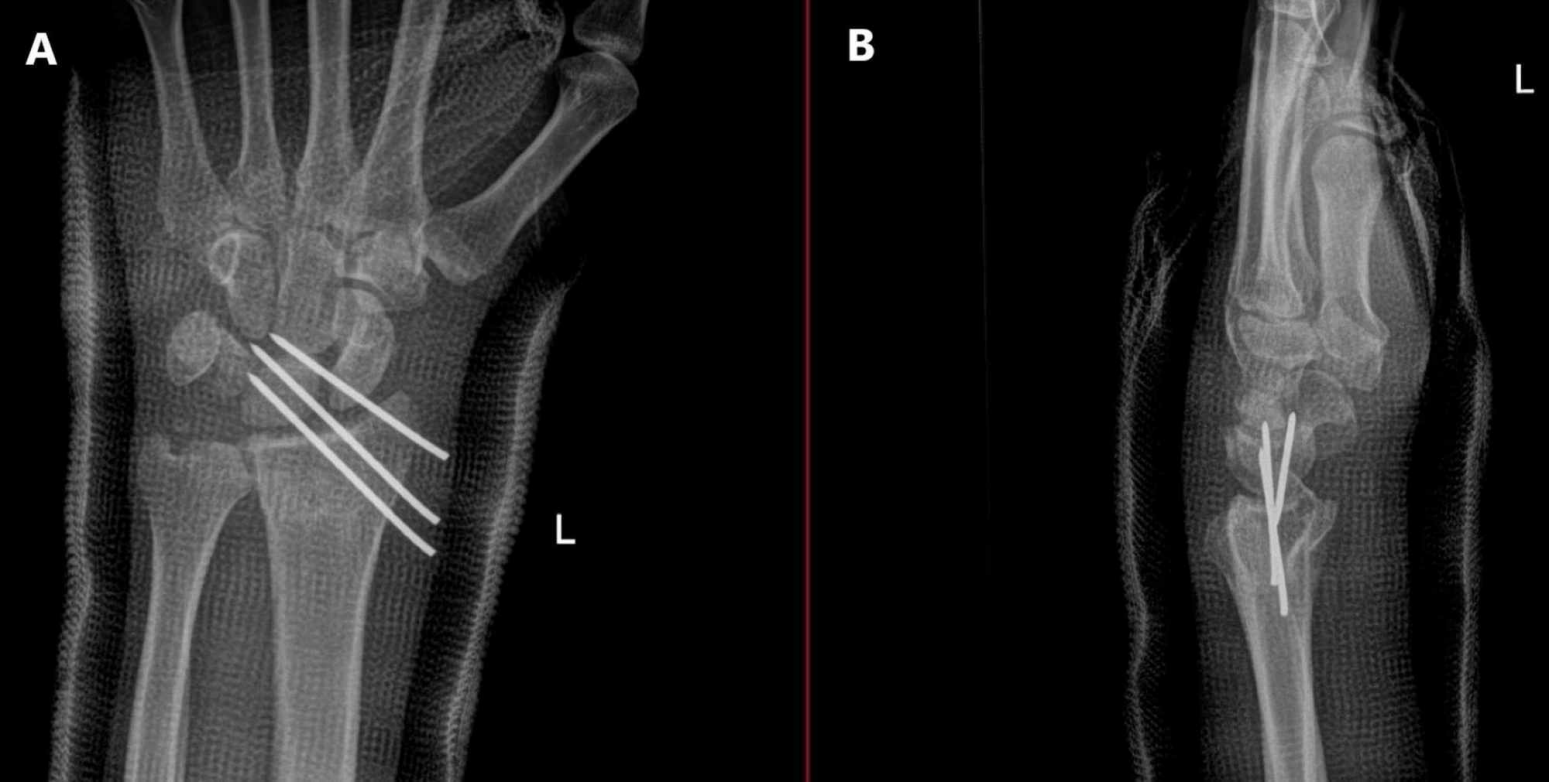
Intraoperative X-ray of the left wrist. A) Anteroposterior (AP) view; B) Lateral view
